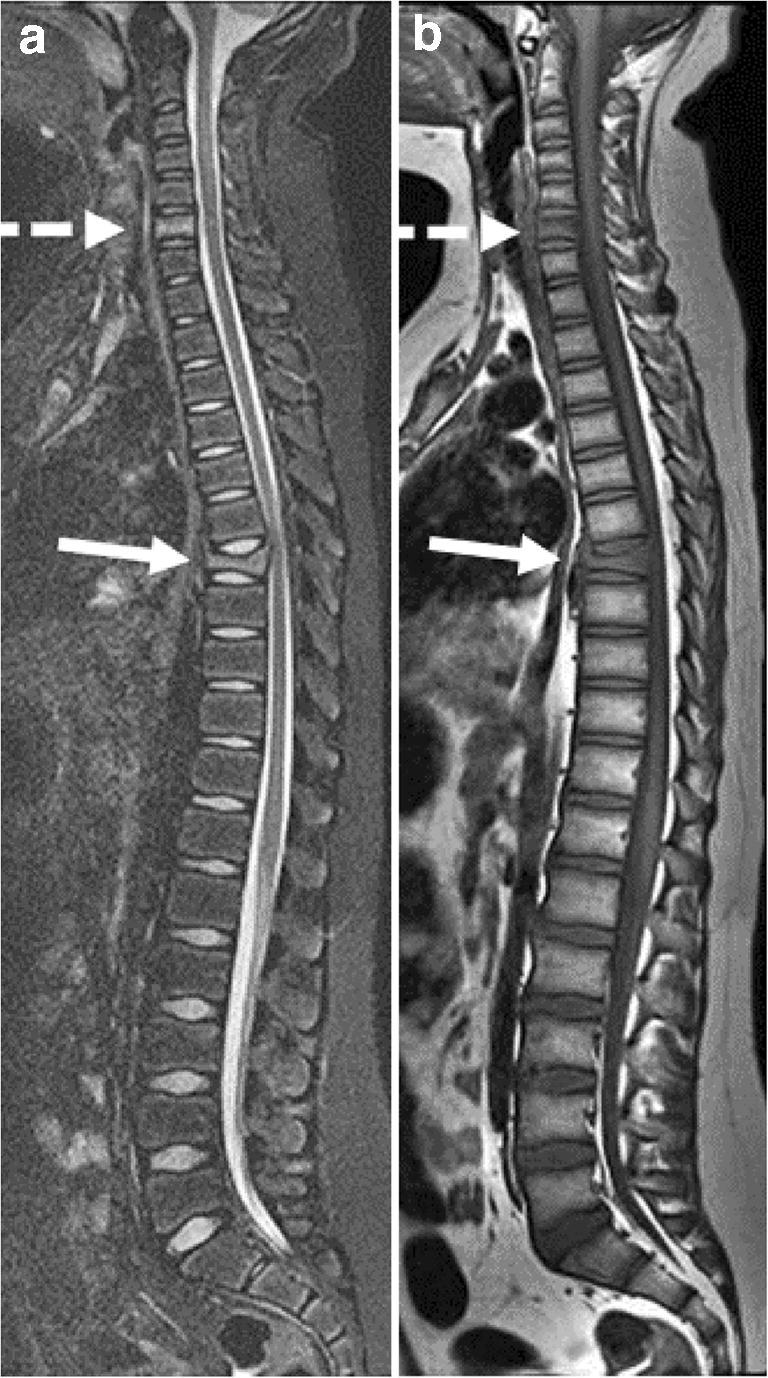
Chronic nonbacterial osteomyelitis in a 13-year-old boy. a, b Sagittal T2-W short tau inversion recovery MR image (repetition time/echo time [TR/TE] 5,000/58 ms) (a) and T1-W MR image (TR/TE 400/7 ms) (b) of the spine show signal change and vertebral compression of the 7th thoracic vertebrae (*solid arrow*) and signal changes of the 6th cervical vertebrae (*dashed arrow*)
